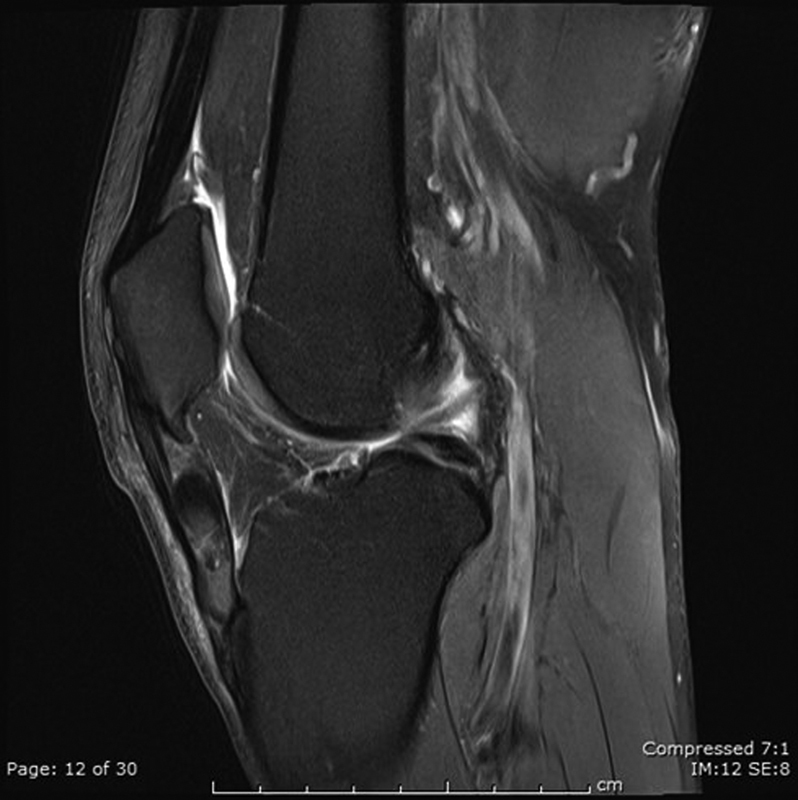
Sagittal magnetic resonance imaging fat suppressed image.
